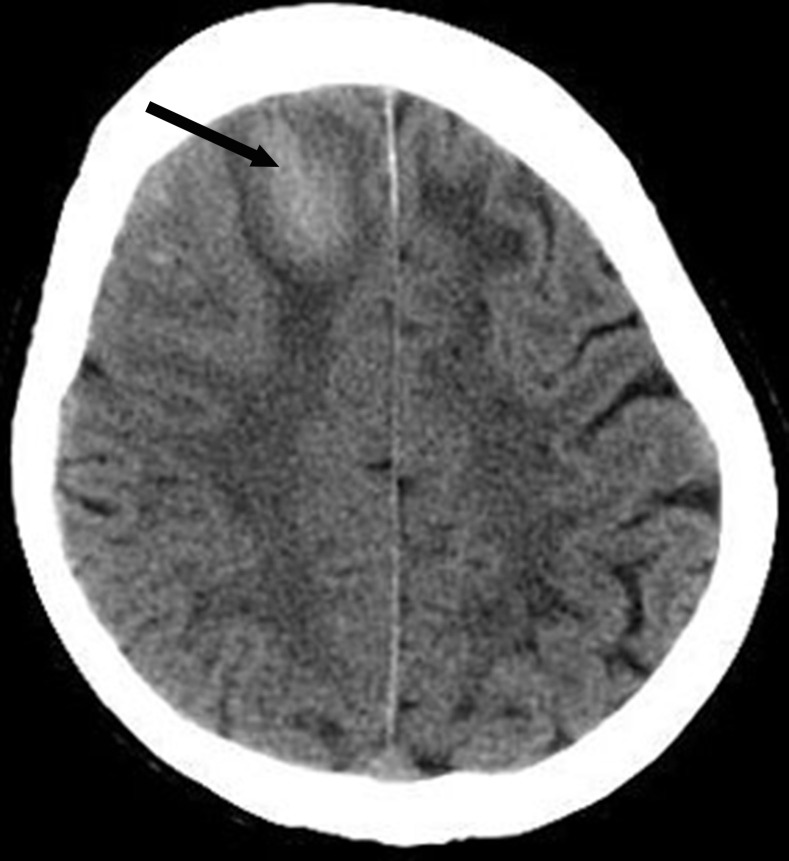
Noncontrast brain computed tomography shows a hematoma (arrow) in the right frontal lobe
